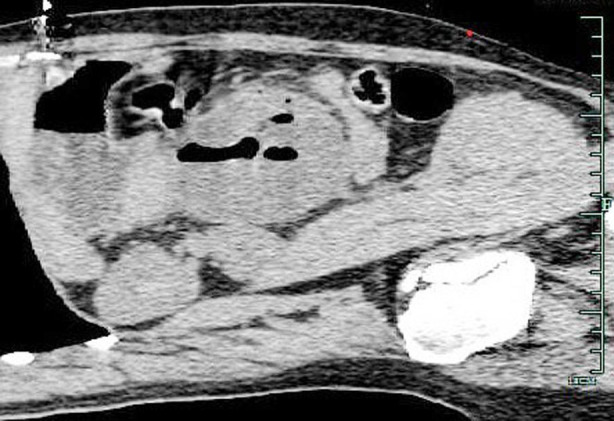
Sagittal CT image of the abdomen shows herniated small bowel with air-fluid levels.
